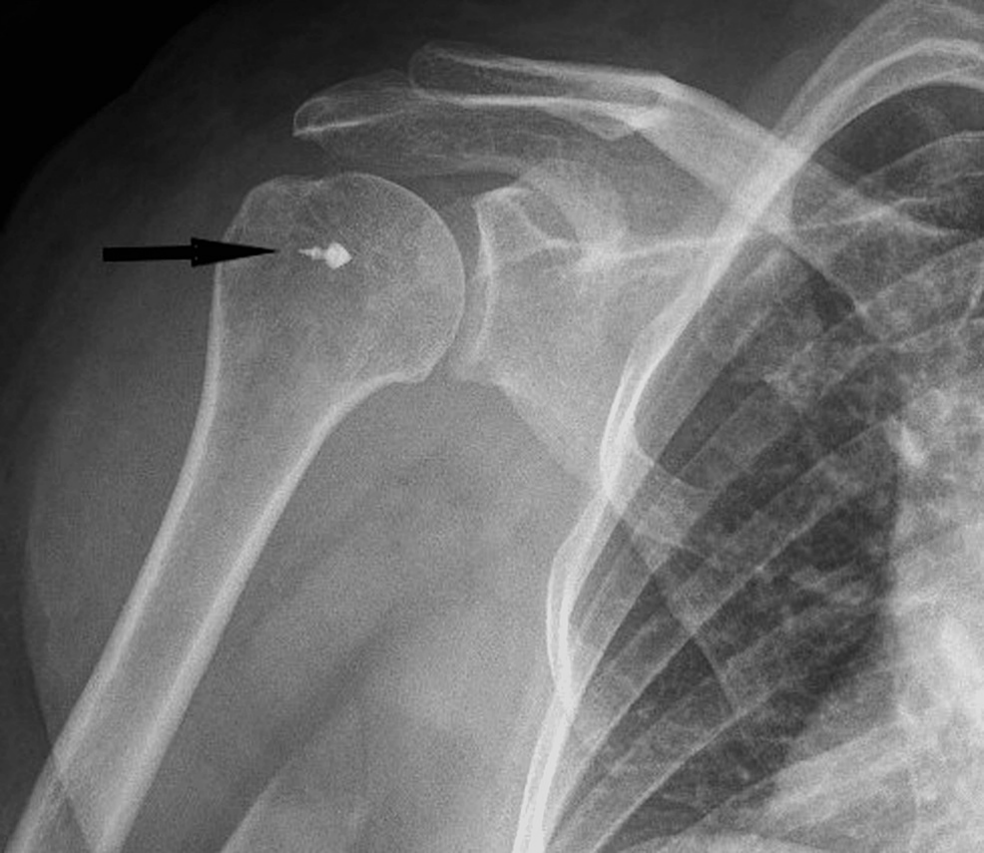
Postoperative plain radiograph of the shoulder showing a bullet tip (of lateral row anchor) in situ (black arrow).
